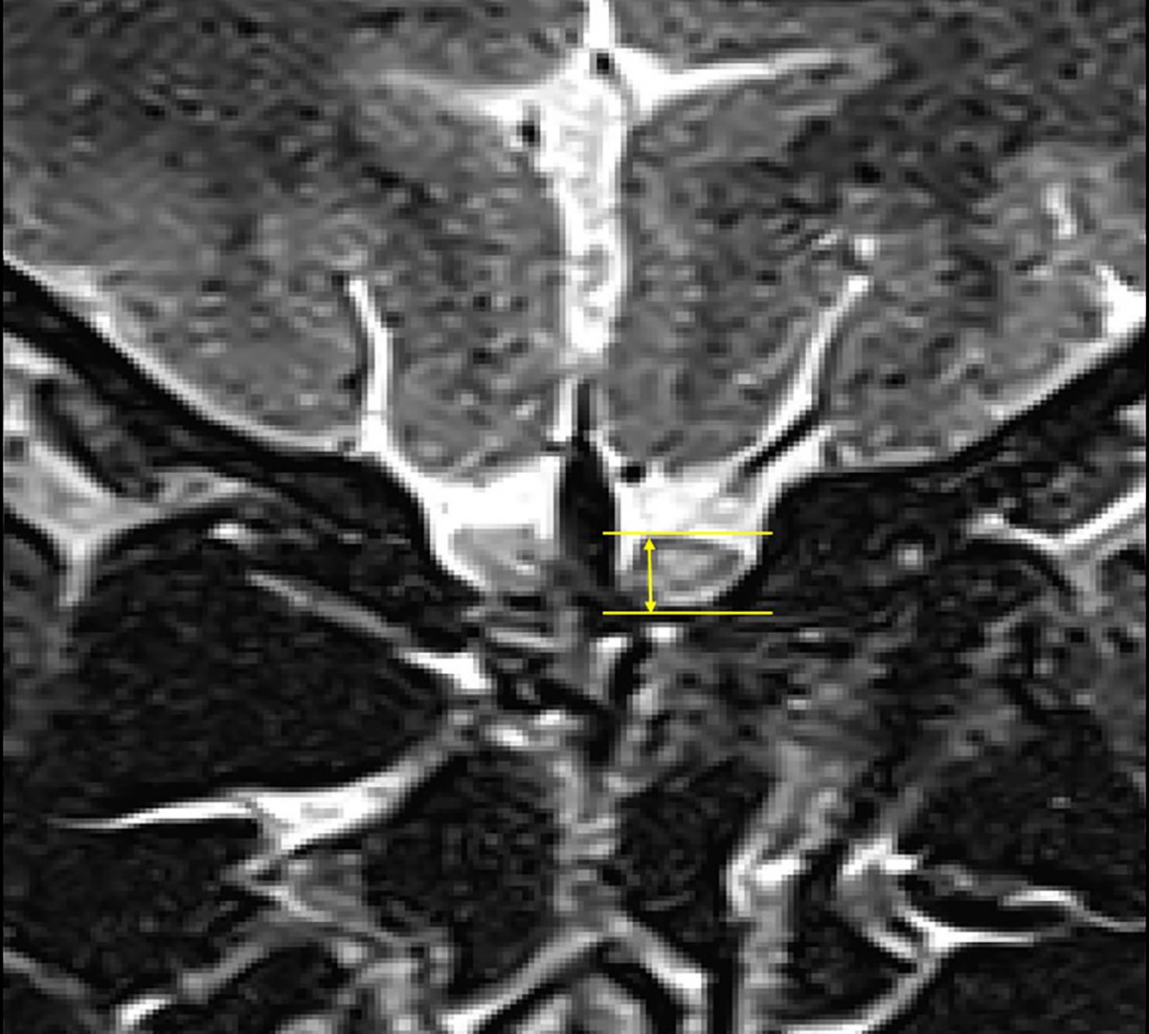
How to measure. A 31-year-old female patient who was diagnosed with drug-induced parkinsonism. Coronal T2-weighted imaging was used to measure olfactory bulb height.
